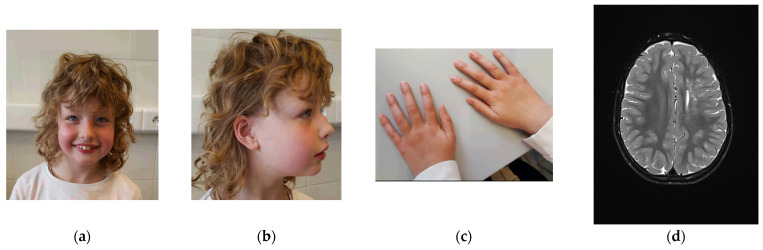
Physical examination at age 7 years and 4 months. (a) Facial features include upslanting palpebral fissures, light blue irises, epicanthal folds, fullness of the upper eyelids, a tubular nose with broad nose tip, and hypoplastic alae nares. (b) Side view: small auricular tag on the right ear and large ear lobes. (c) Picture of the hands: slender hands with rather long fingers, and slightly increased skin wrinkling. (d) Brain magnetic resonance imaging (MRI) at age 6 years and 9 months shows an increased T2 signal in the corona radiata on the left side. Furthermore, limited ex vacuo dilatation of the left lateral ventricle and atrophy of the anterior segment of the corpus callosum are present.
